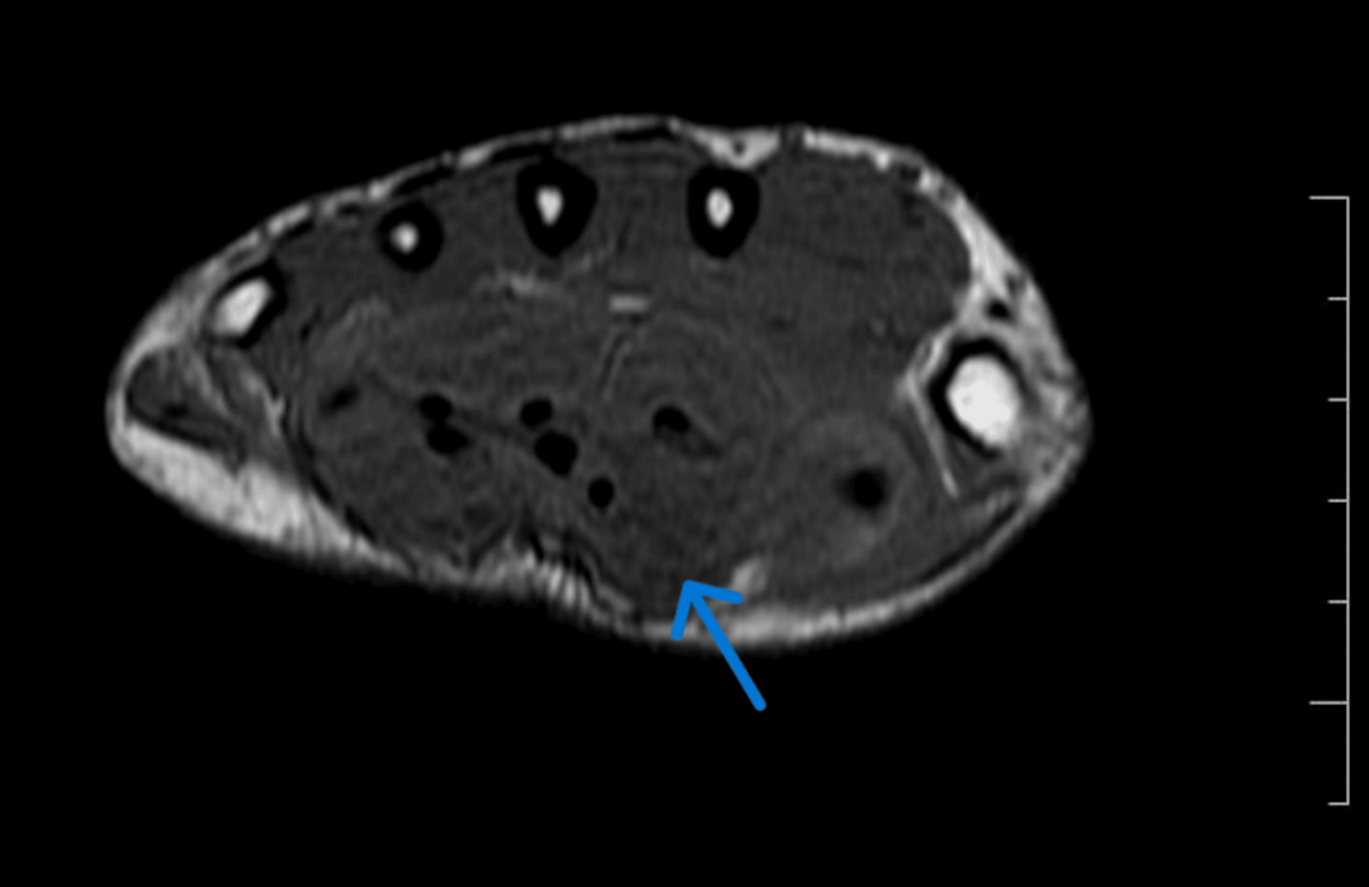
Axial T1-weighted MR image distal to the carpal tunnel demonstrates distended radial and ulnar bursae with numerous isointense corpuscles (rice bodies)
